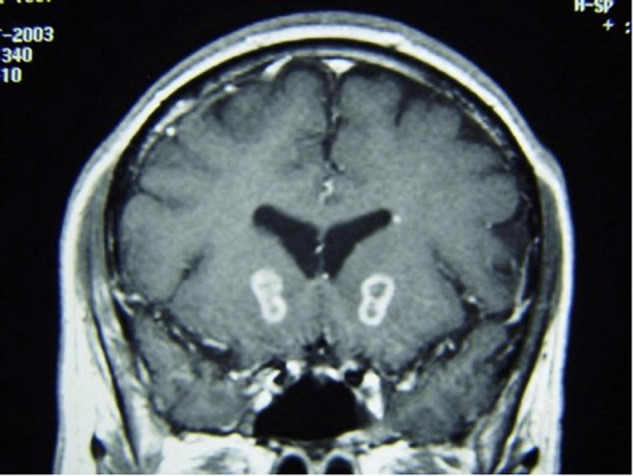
Coronal T1-weighted MRI with contrast of gamma knife capsulotomy lesions.
